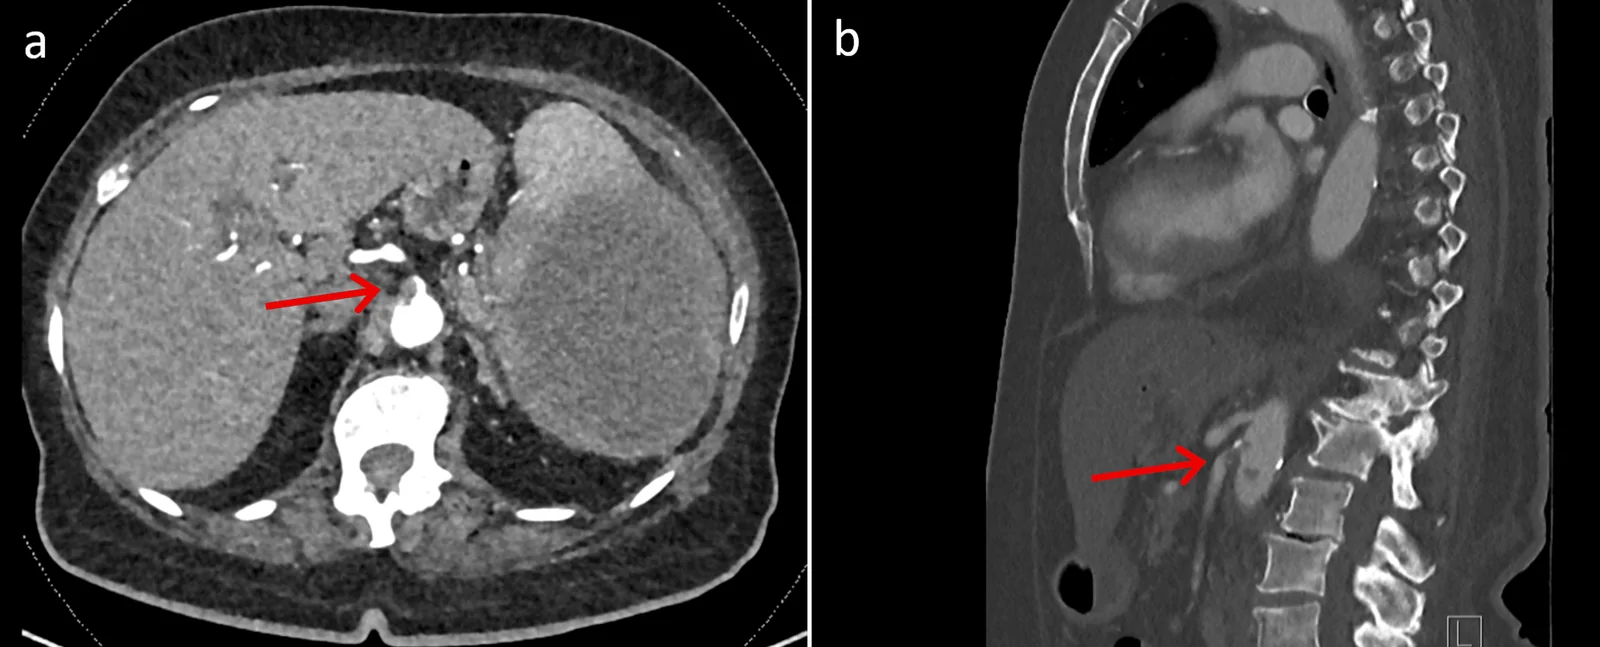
Transversal (a) and sagittal (b) section of the thoraco-abdominal CT scan in portal phase; atherosclerotic plaque at the ostium of the superior mesenteric artery indicated by arrows
